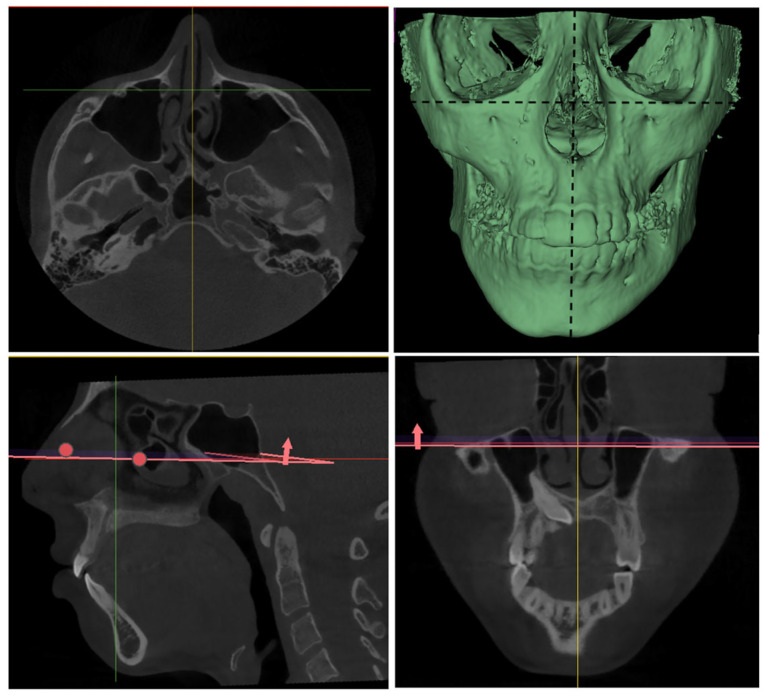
CBCT images were oriented with the Frankfurt Horizontal (FH) plane parallel to the ground and properly aligned with the midsagittal plane.
